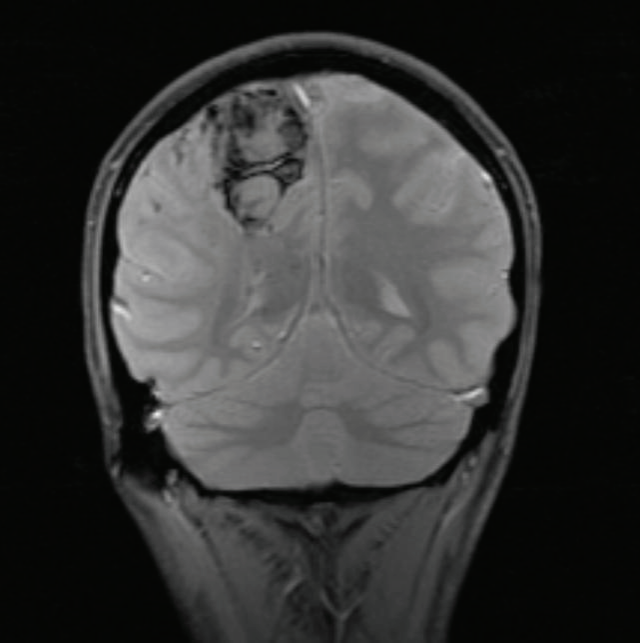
Brain cavernomas. Coronal cut MRI image of the brain cavernomas located ipsilateral and directly beneath the en coup de sabre linear scleroderma lesion of case #1.
